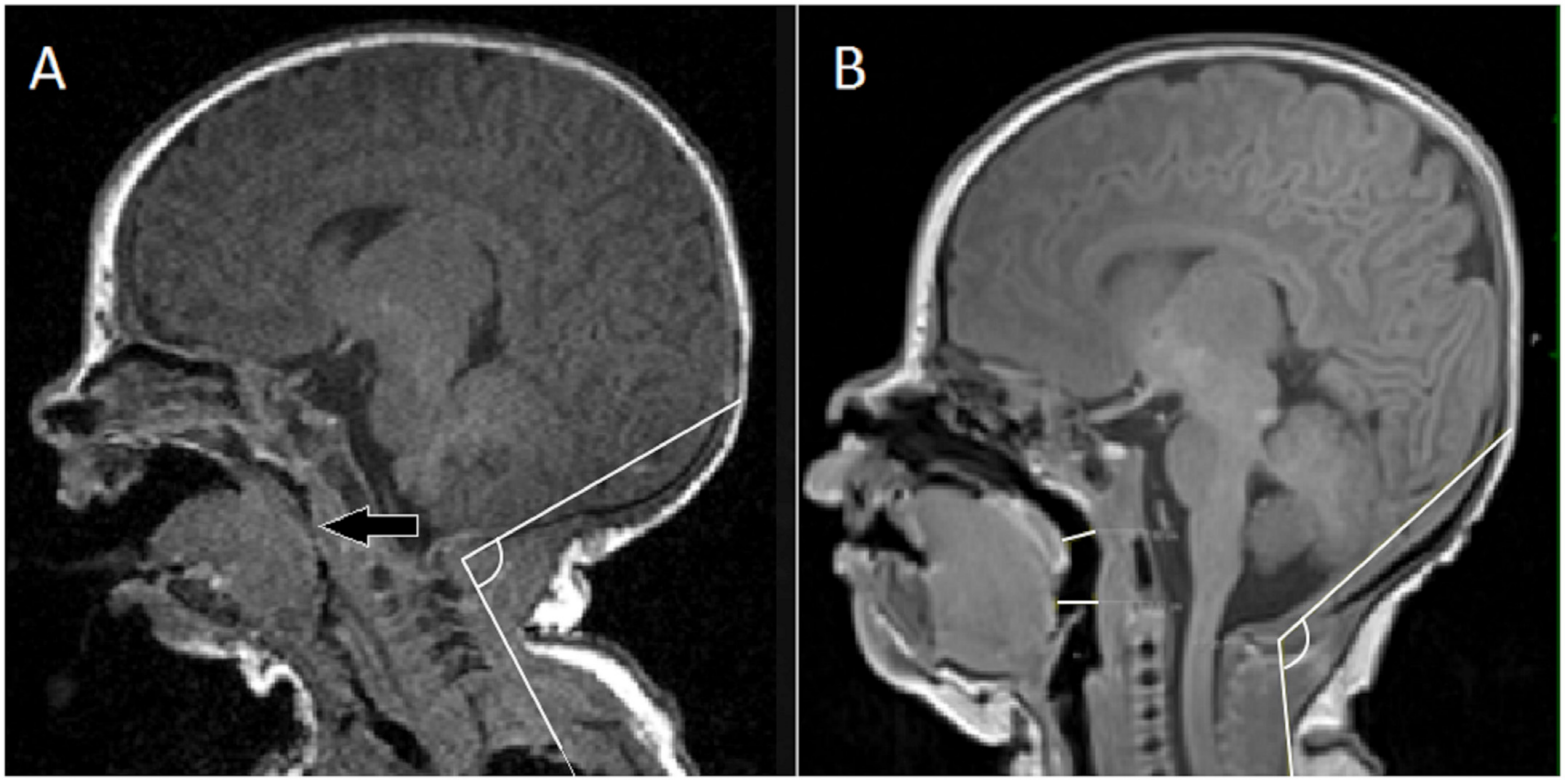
A—Sagittal T1-weighted MR image of head-neck region of a newborn-infant showing obstructed airway at the tongue level. B—Sagittal T1-weighted MR image of head-neck region of a newborn-infant showing a patent airway.
